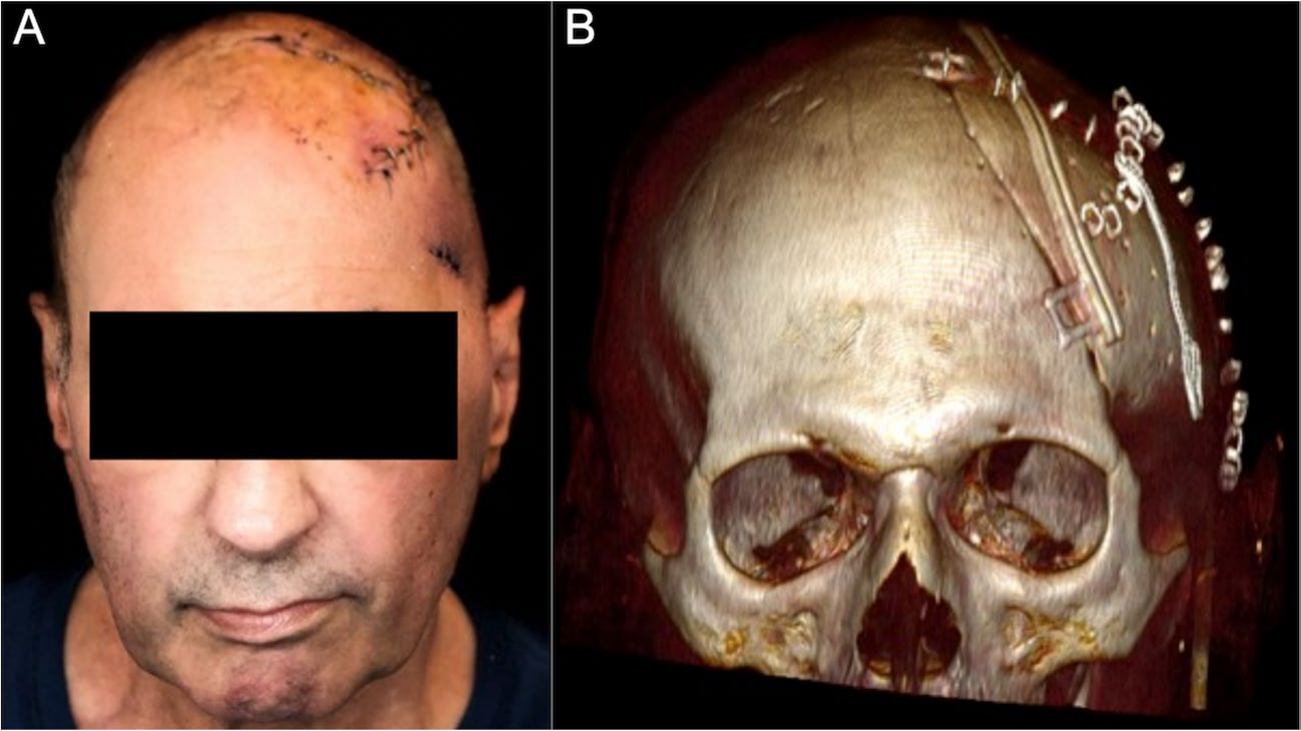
Censored photograph 1 week postoperatively after 3D printer-assisted cranioplasty of a frontotemporoparietal defect (A) and 3D reconstruction based on CT imaging showing an accurate symmetric cranial contour (B)
